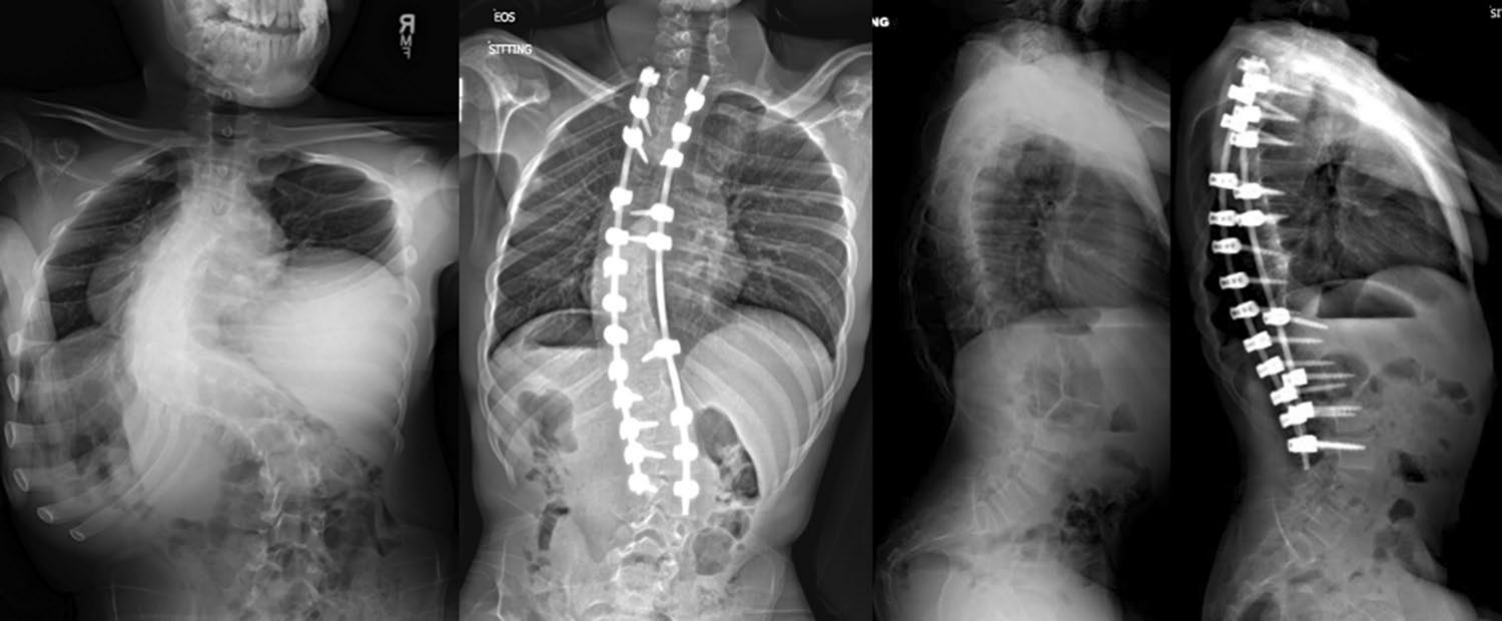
Thirteen-year-old male with neuromuscular scoliosis (who is ambulatory) measuring 106 degrees with C-DAR = 13, S-DAR = 9, and T-DAR = 22. This patient underwent PSF from T2-L3 without vertebral column resection or three-column osteotomy. This patient had loss of IONM signals but did not develop a post-operative neurological deficit. Radiographs at 6 months postop demonstrate significant deformity correction
